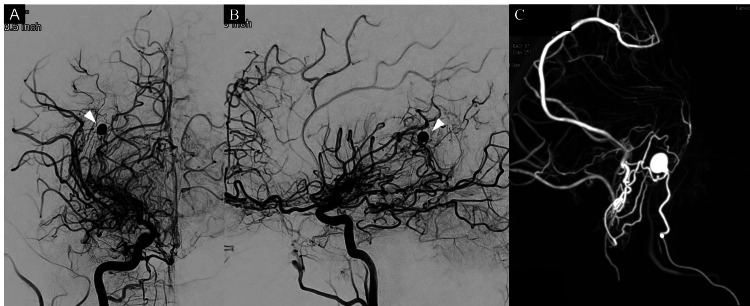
Angiography prior to embolization. Frontal (A) and lateral (B) views of the right internal carotid artery angiography showed an aneurysm in the peripheral anterior choroidal artery (white arrowheads). Compared to the angiography performed in the acute phase, the aneurysm had neither shrunk nor disappeared. Super-selective contrast cone-beam computed tomography revealed a 6 mm aneurysm and ventriculofugal collateral supply to the right posterior parietal lobe (C).
